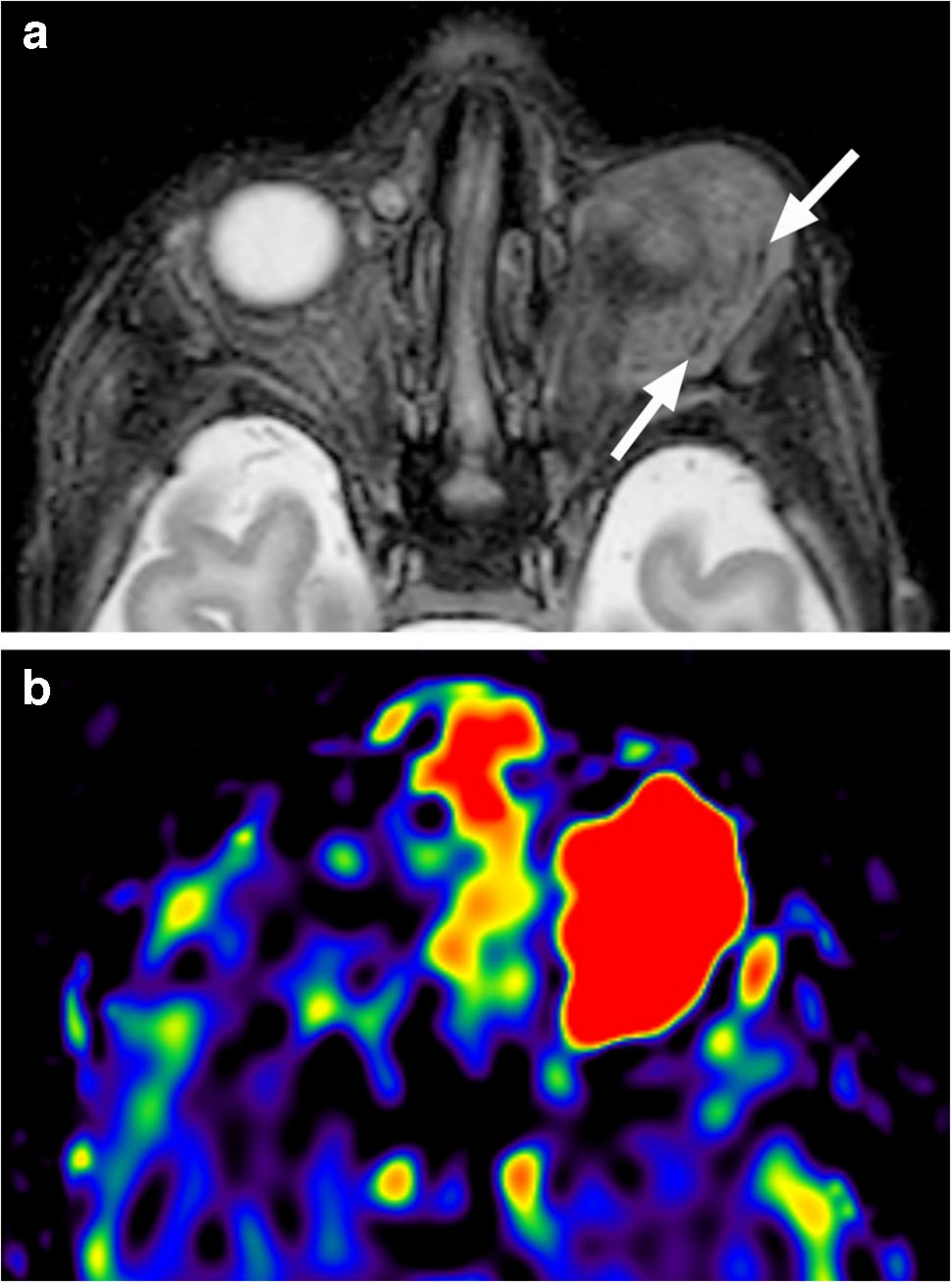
Axial T2-weighted image of the orbits (a) shows a preseptal and postseptal intraorbital lesion containing flow voids (arrows), which demonstrates intense homogenous hyperperfusion with arterial spin labeling (ASL) (b). The lesion also demonstrated prominent homogenous enhancement and high ADC values, hyperintense to the brain (not shown), consistent with infantile hemangioma
